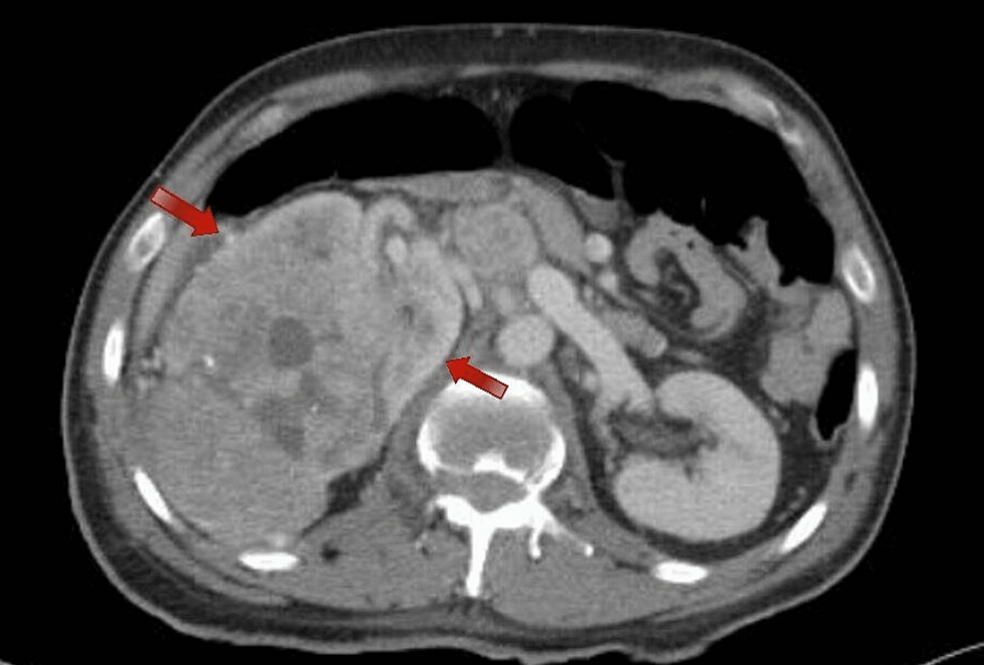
Axial view CT Abdomen with IV contrast enhancement demonstrating right renal mass
